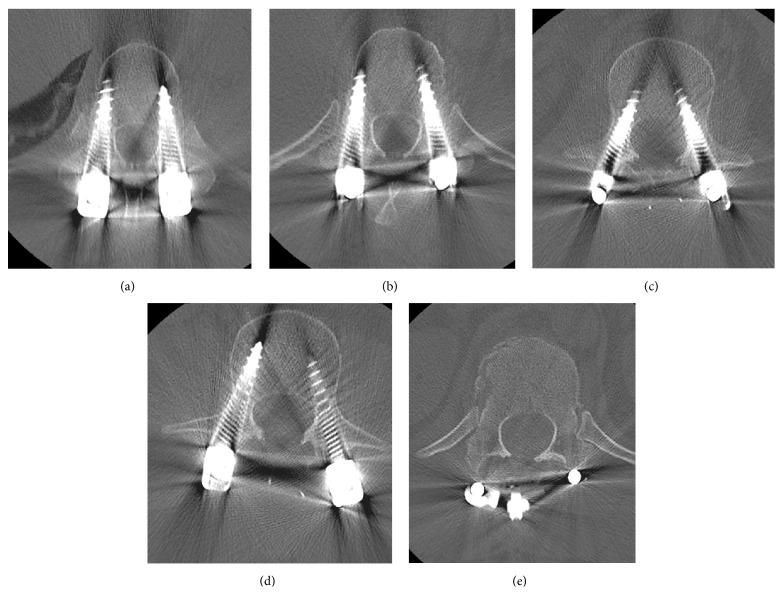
Postoperative CT images of the spine. Axial image (a) T10, (b) T11, (c) L1, (d) L2, and (e) T12. All pedicle screws from T10 to L2 were in correct position, and there were no protruding fracture fragments in the spinal canal at the T12 level from reduction of the dislocated fracture during the operation.
